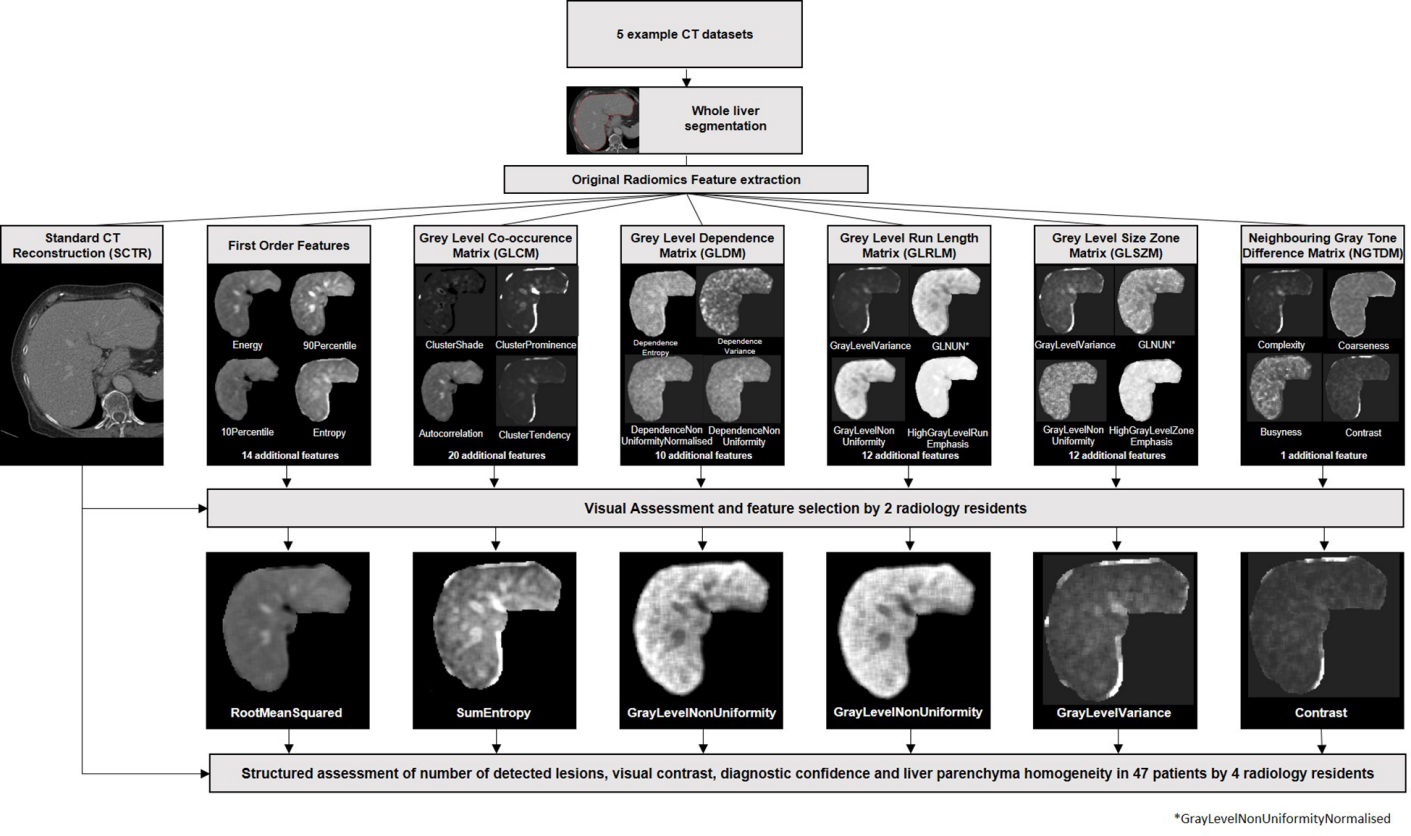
Study protocol. Whole liver segmentations were performed in five example CT datasets. Standard radiomics features were extracted and visualized parameter maps. Based on visual assessment by two readers, one feature of each feature class was selected. Subsequently, all parameter maps as well as the standard CT reconstructions of all patients were evaluated by four readers in a structured manner and evaluated according to the number of lesion, visual contrast, diagnostic confidence, and liver parenchyma homogeneity.
